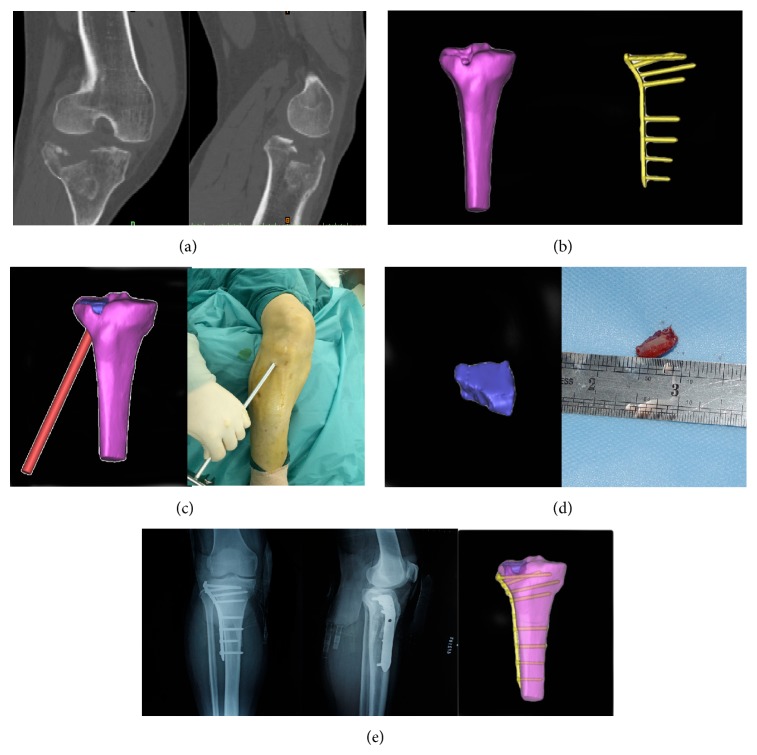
Comparison of virtual and actual surgeries. (a) Actual CT scan of a tibial plateau fracture. (b) Three-dimensional models of the same tibial plateau fracture and its internal fixation. (c) Creation of a surgical windowing and elevating reduction in the virtual and actual surgeries. Window position, window angle, and effective reduction were identical in the virtual and actual surgeries. (d) Virtual and actual bone grafts. (e) Postoperative radiographs were consistent with the virtual surgery and internal fixation.
